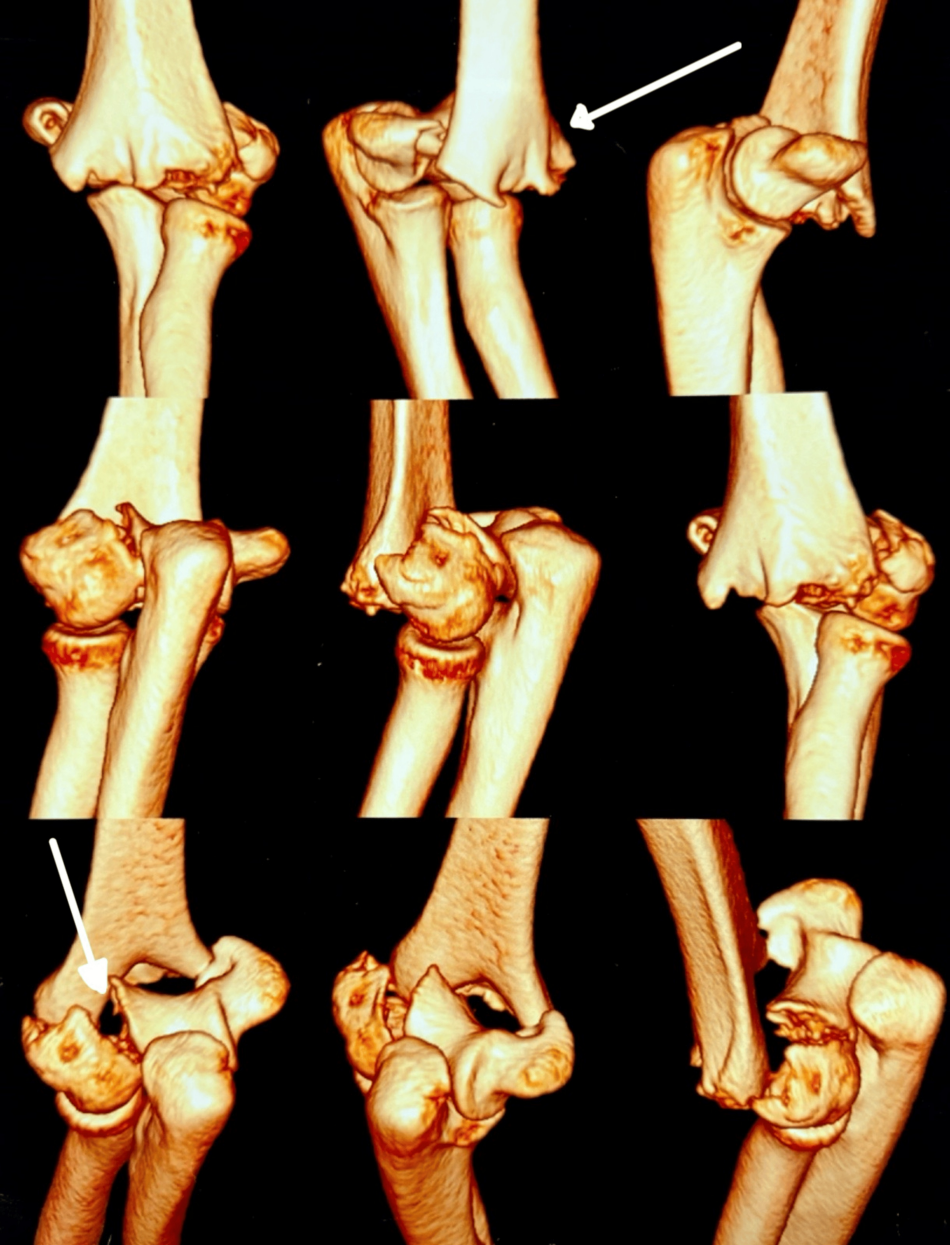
Three-dimensional computed tomography of the left elbow showing a distal humerus intra-articular fracture
